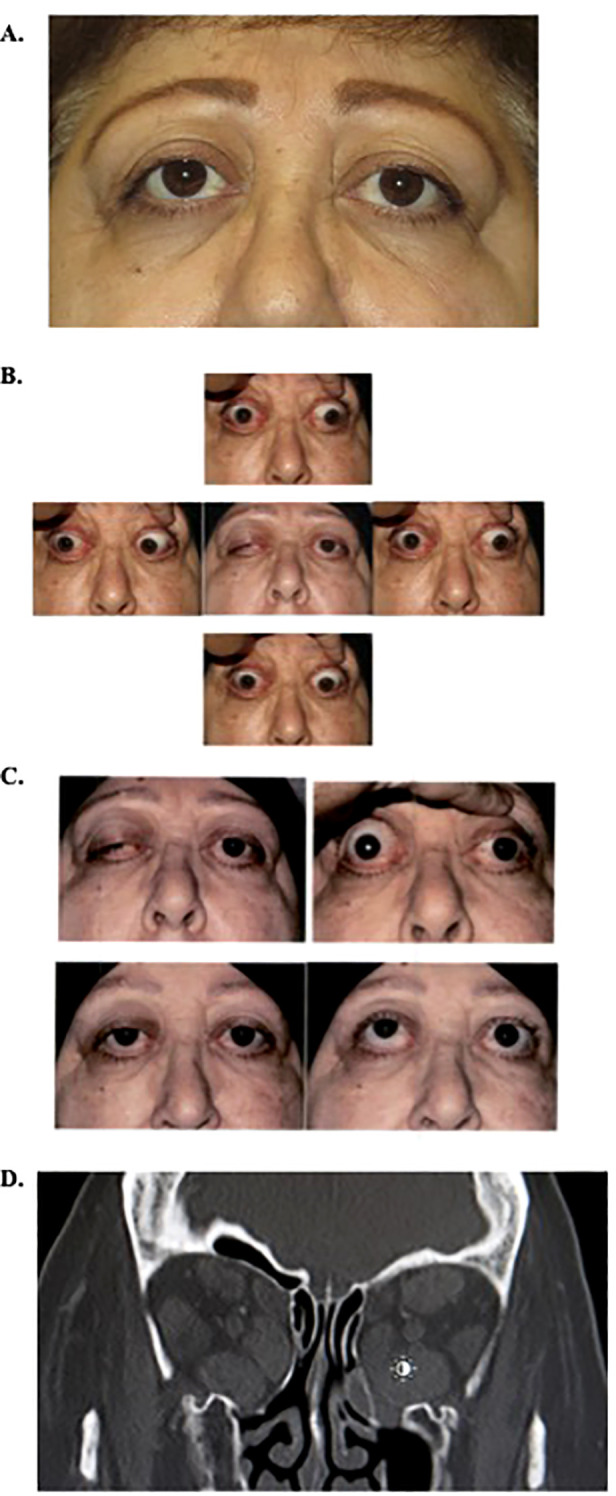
(A) External photo prior to the current episode of TED reactivation, after bilateral graded transantral decompression in 2004, Clinical Activity Score of 0. (B) External photos on initial presentation showing reactivation of TED demonstrating ptosis, proptosis, and decreased extraocular motility in all fields of gaze. (C) External photos before and 4 weeks after initial presentation following IV methylprednisolone, one dose of teprotumumab, and two doses of low-dose orbital radiation. Photos in primary and upgaze. (D). Coronal image, computed tomography of orbits on current presentation, demonstrating enlargement of extra-ocular muscles. Note previous graded transantral orbital decompression with infracture of medial wall and orbital floor removal. TED, thyroid eye disease.
